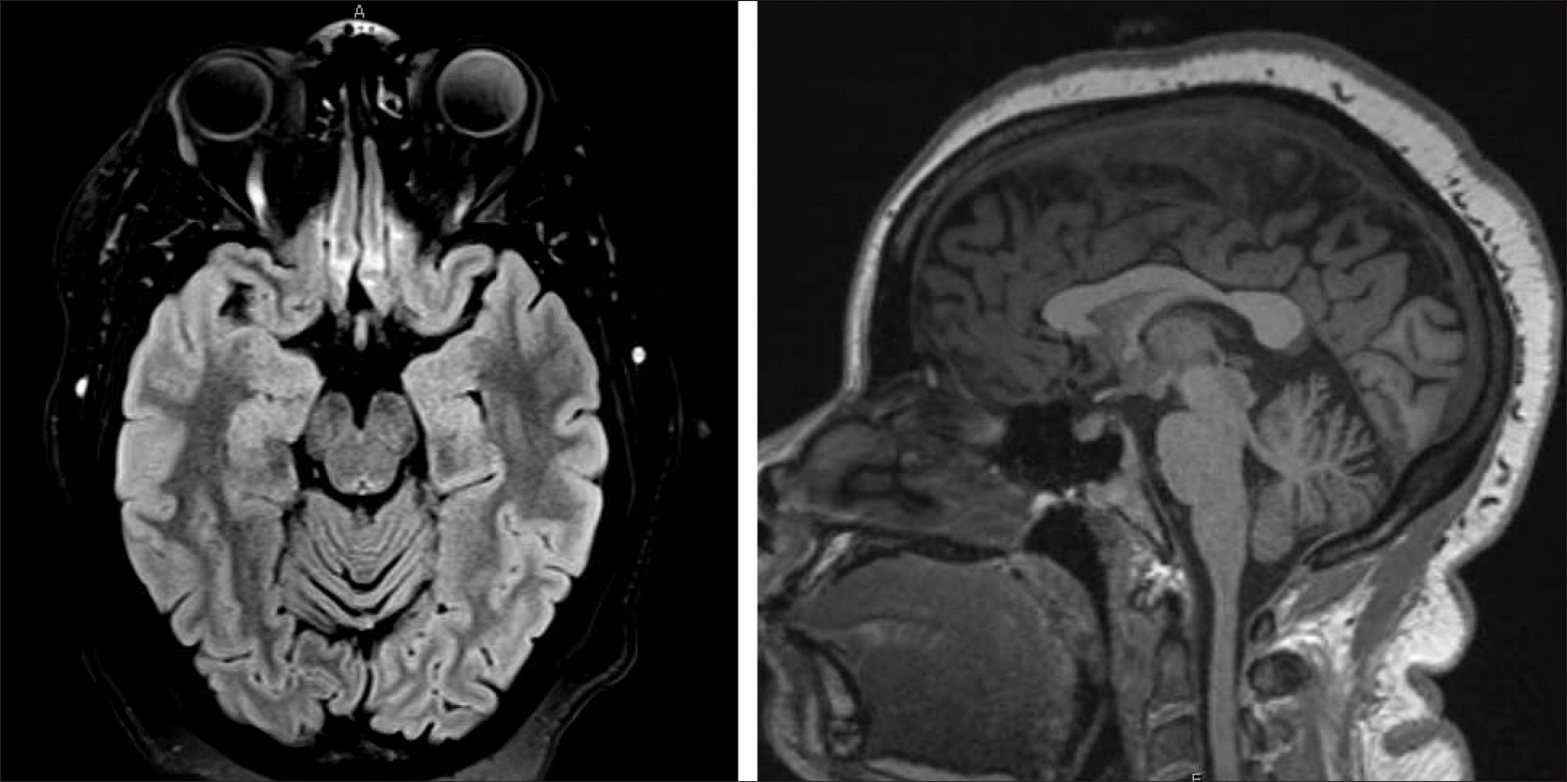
Brain MRI of the described case. The images show axial FLAIR (on the right) and coronal T1 (on the left) brain MRI imaging of this case, at age 25. Both images showed a mild cerebellar atrophy.
